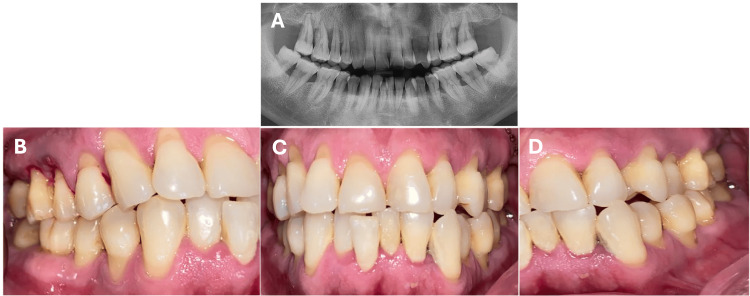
Initial clinical presentation of the patient diagnosed with stage IV, grade C, generalized periodontitis. (A) Panoramic radiograph taken prior to the start of periodontal treatment, showing severe generalized bone loss around the teeth. (B) Right lateral photograph, (C) left lateral photograph, and (D) frontal view showing generalized inflamed periodontal tissues, with multiple gingival recessions, gingival bleeding, dental crowding, the presence of dental calculus, and gingival erythema.
